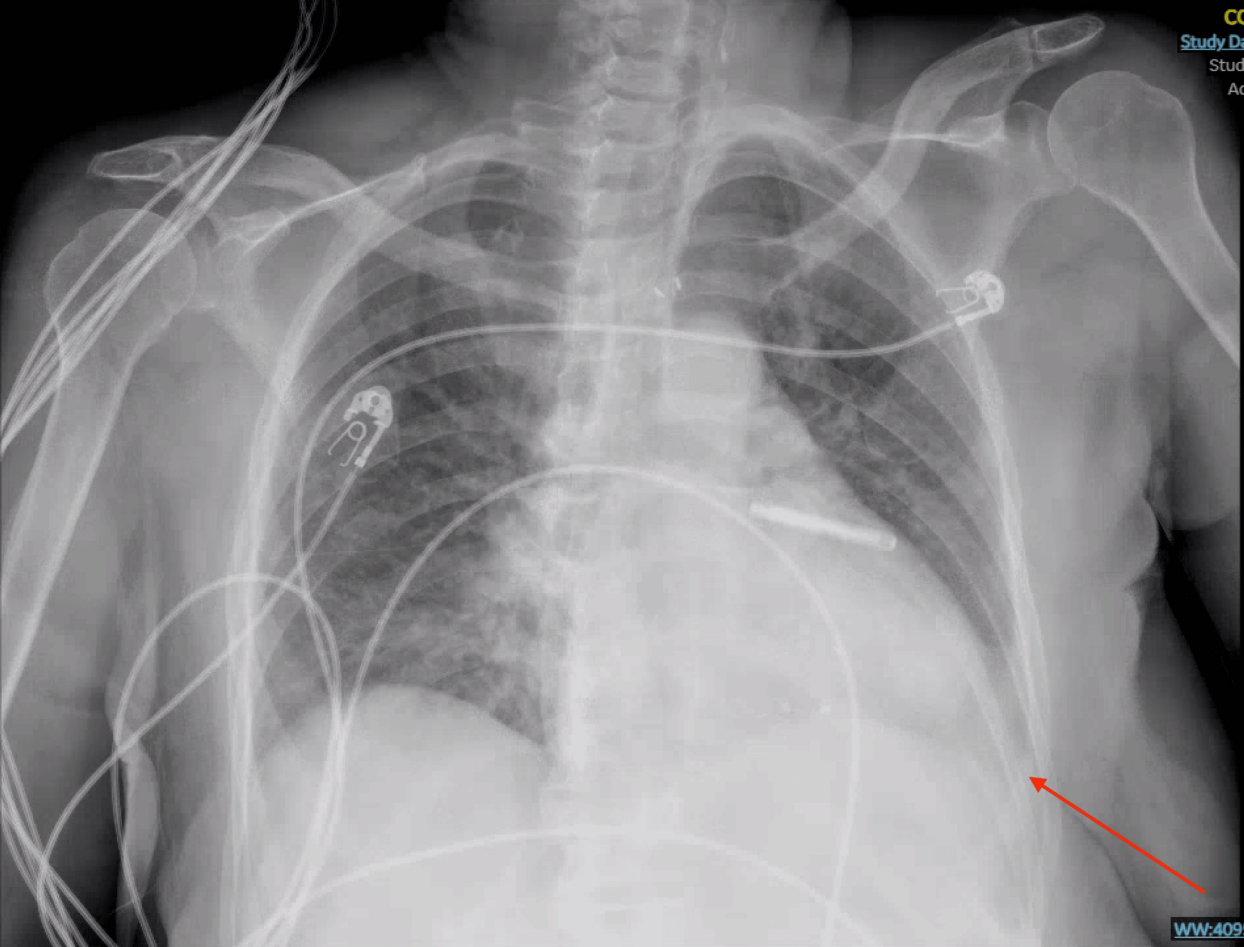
Chest X-ray on hospital day one showing left-sided pleural effusion (red arrow).
